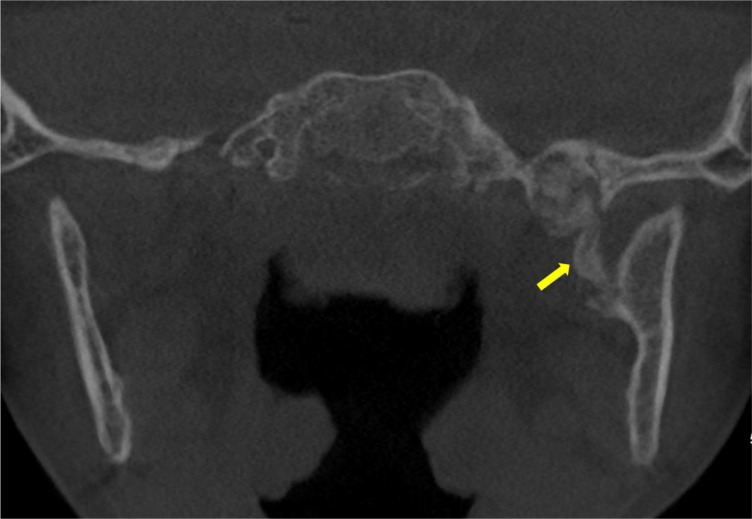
Unenhanced CT scan showing an ossified structure located between the left mandibular ramus and the spine the left sphenoid (arrow).
